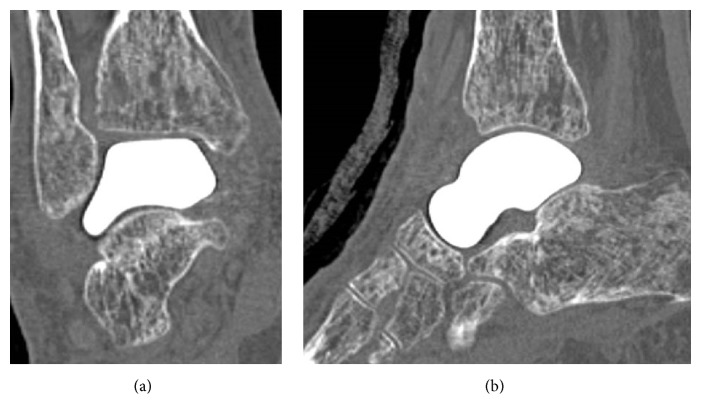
Postoperative CT scans showing that the prosthesis and surrounding bones are well aligned in coronal (a) and sagittal (b) views at 1 year and 6 months after surgery. There is no osteolytic change in the surrounding bone or sinking of the prosthesis.
